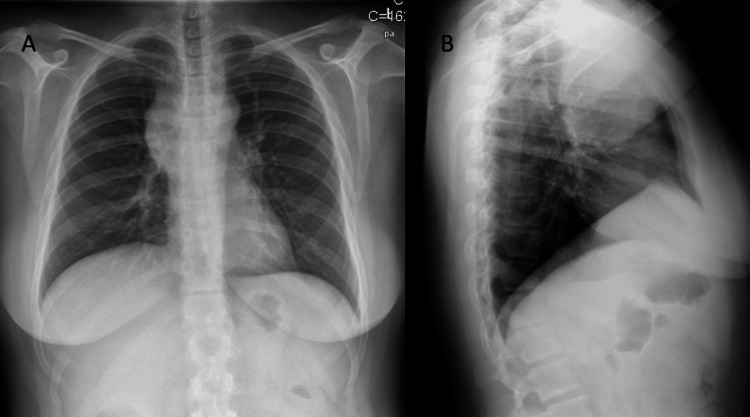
Posteroanterior (A) and lateral (B) thorax radiography showing enlargement of the mediastinum (lobulated mass of 9.0 cm).
